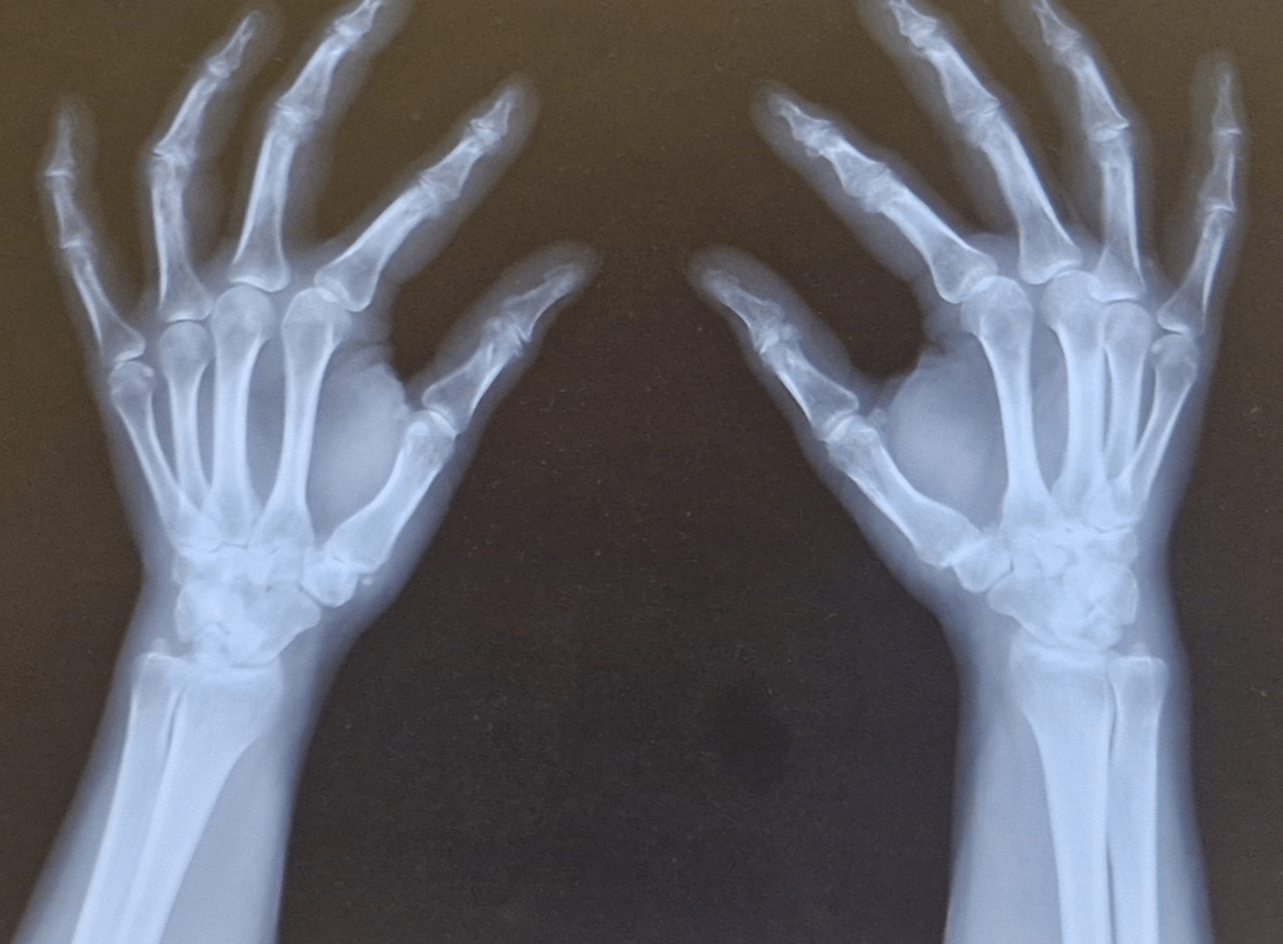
Preoperative X-ray in the Kapandji projection
